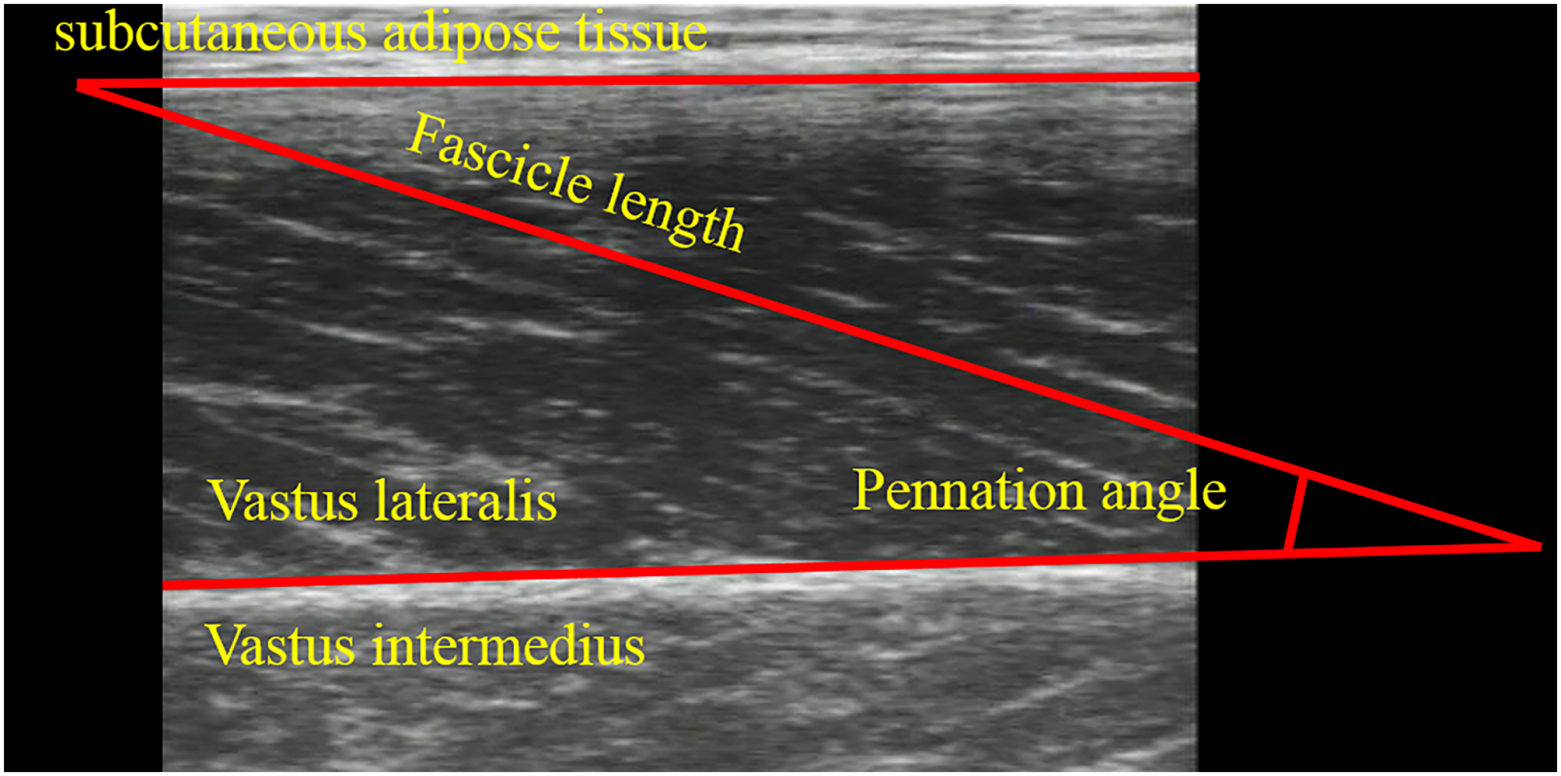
Ultrasonographic image of the vastus lateralis.
